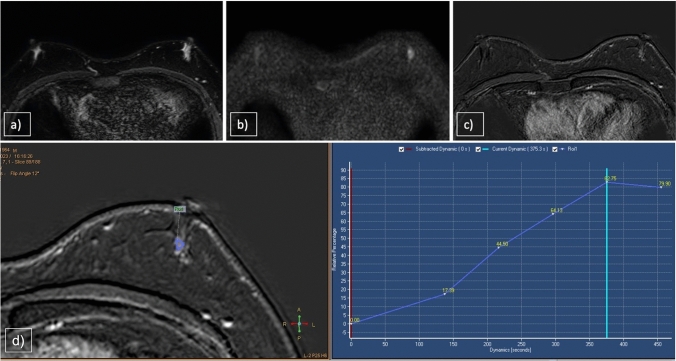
a STIR, b DWI, c CE T1WI, d Time–Intensity Curve. 32-year-old patient with bloody nipple discharge on the left. The MRI showed bilateral gynecomastia with restricted signal in DWI on the left (a,b,c). Subareolar non-mass enhancement with a type 1 time–intensity curve was evident (d). Biopsy confirmed malignant nature of the lesion (DCIS)
